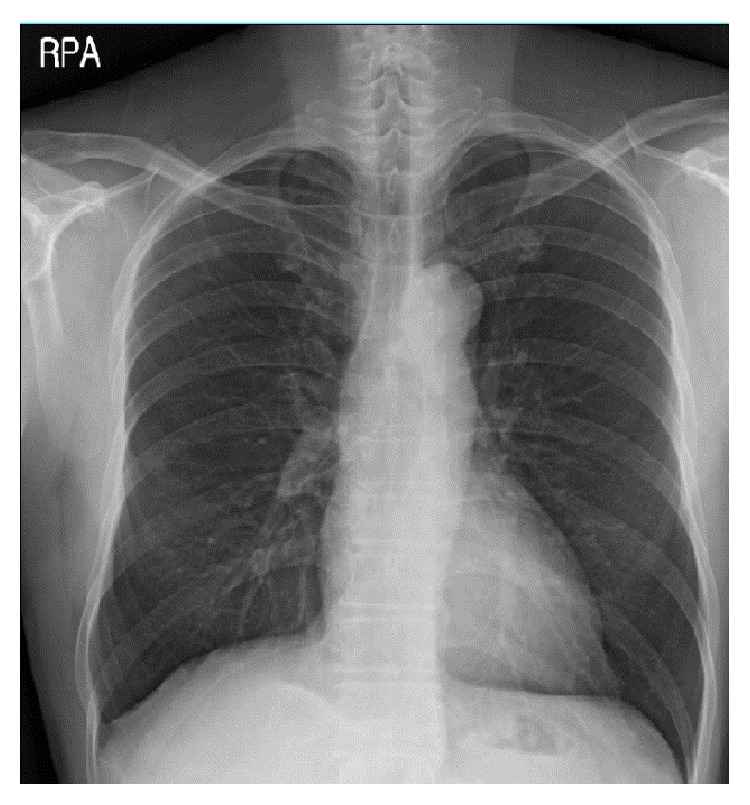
A chest radiograph showed the complete resolution of empyema without pleural sequelae 12 months after discontinuing treatment.
